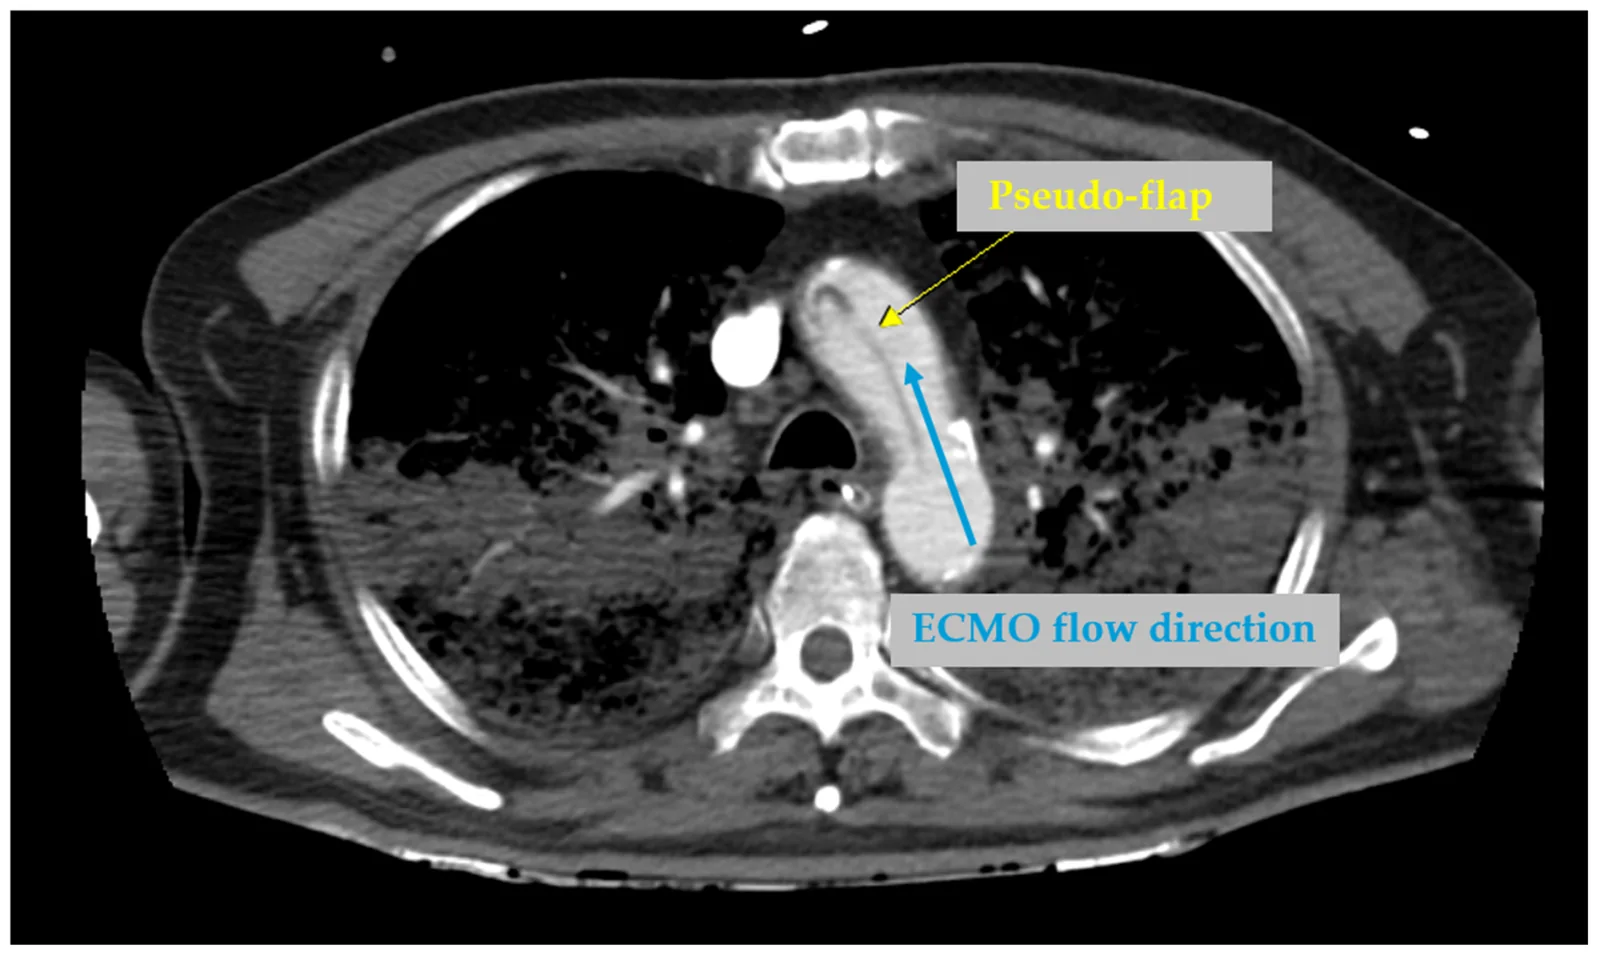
CTA artifact mimicking acute aortic dissection. Axial view reveals a pseudo-flap in the aortic arch (yellow arrow) generated by fluid dynamic interference. Retrograde, unenhanced flow from peripheral VA-ECMO (blue arrow) collides with enhanced, native cardiac ejection, producing a contrast-layering artifact indistinguishable from a true intimal flap.
